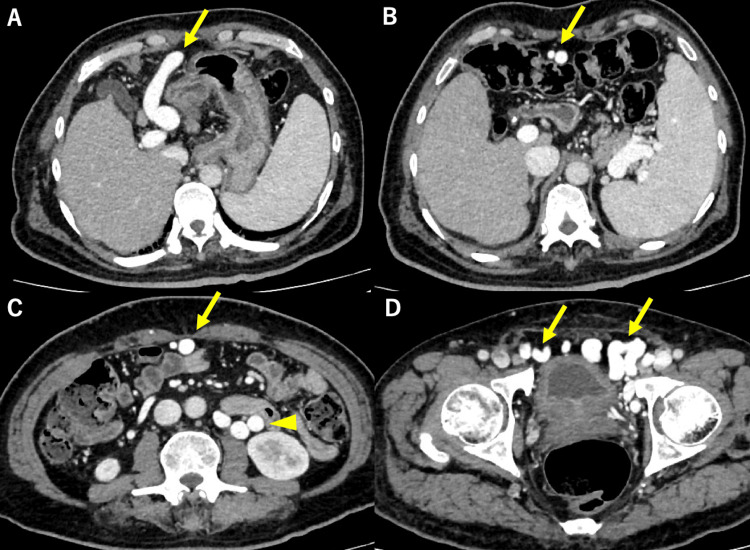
Contrast-enhanced CT of the portal phase. (A) Enlarged paraumbilical vein (yellow arrow) connecting the left branch of the portal vein. Splenomegaly is shown. (B) Paraumbilical vein running through the abdominal wall (yellow arrow). (C) In addition to the paraumbilical vein (yellow arrow), a mesorenal shunt is also seen (yellow arrowhead). (D) Paraumbilical vein connecting the bilateral common femoral vein (two yellow arrows).
